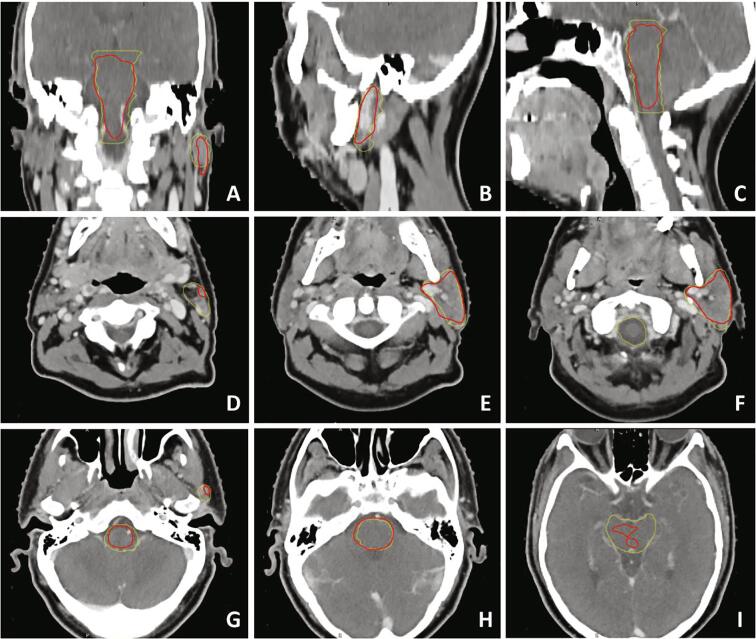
CT slices of an example case, showing differences in performance dependent on craniocaudal location. Contours are displayed for brainstem and left parotid gland, with manual RUMC reference contours given in yellow, and DLC results in red. A: coronal image; B: sagittal image showing left parotid gland contours; C: sagittal image displaying brainstem; D-I: transversal images, with D the most caudal and I the most cranial image. (For interpretation of the references to colour in this figure legend, the reader is referred to the web version of this article.)
